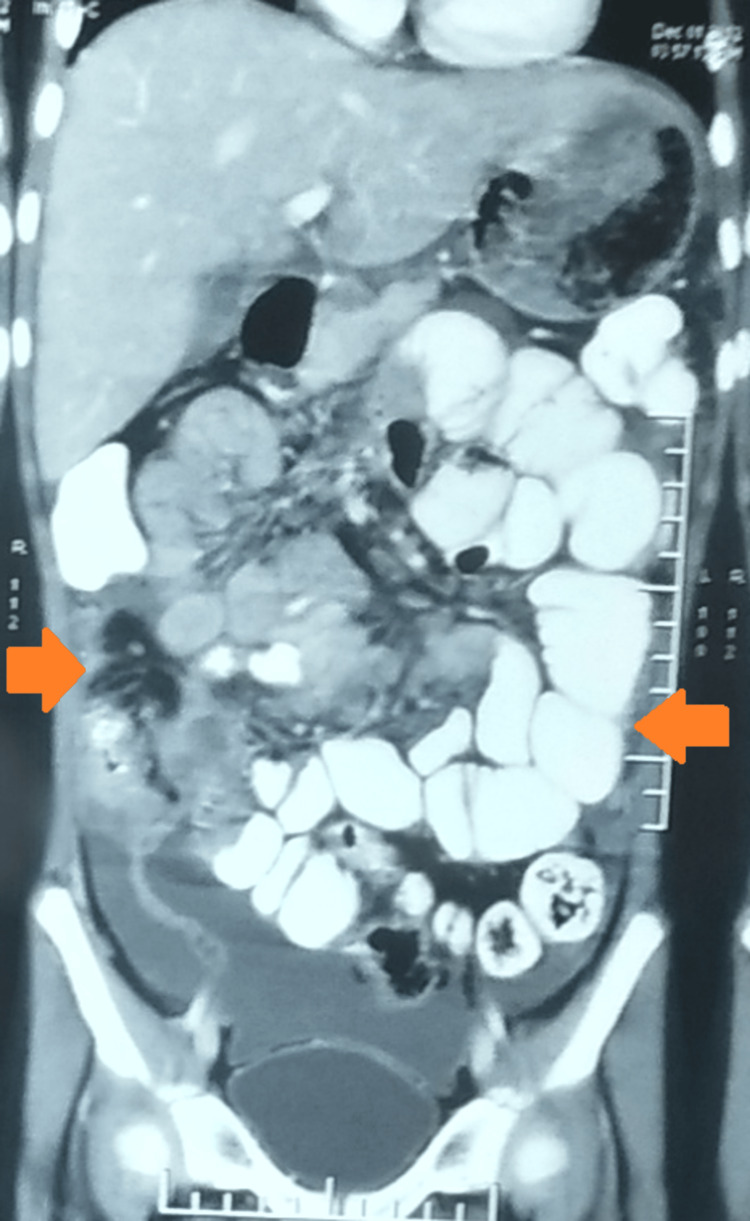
A CECT-whole abdomen showing irregular circumferential thickening involving the terminal ileum, caecum, and part of the proximal ascending colon with multiple discrete enlarged lymph nodes (arrows) CECT: contrast-enhanced computed tomography
